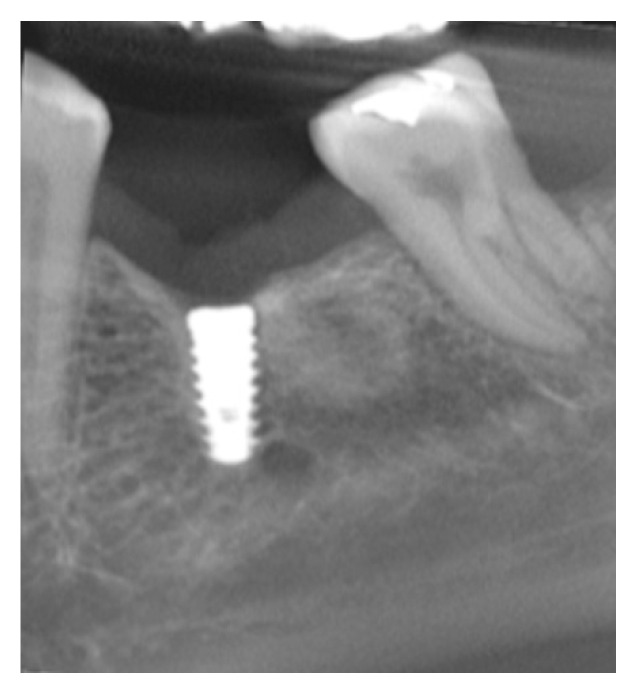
Radiographic image of a fractured abutment.
